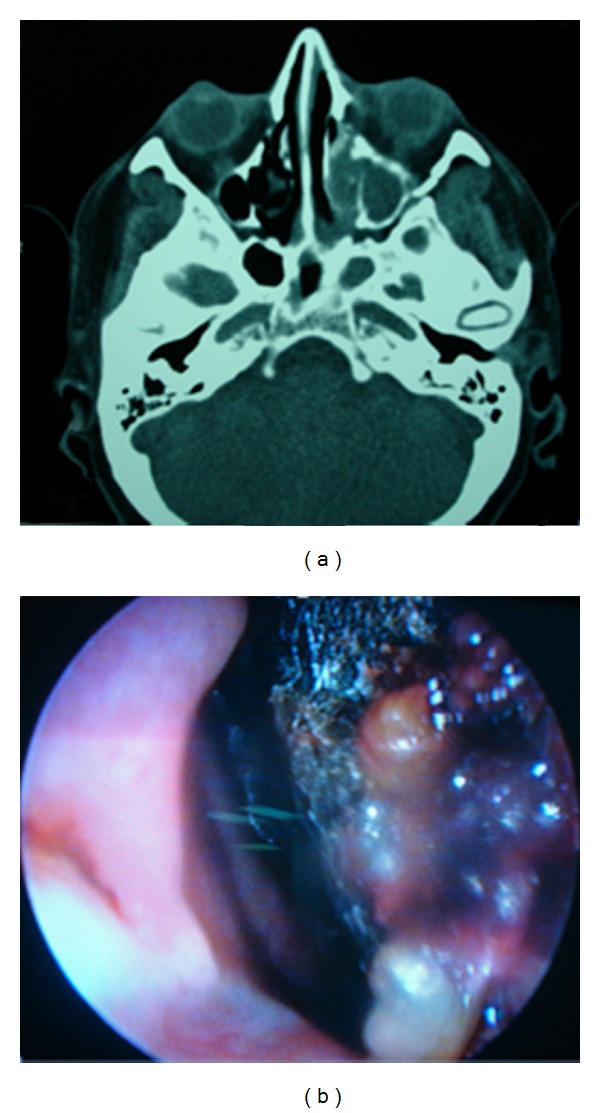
(a) CT scan of patient with invasion of left paranasal sinuses and orbit. (b) Necrotic sinus tissue found on endoscopy.
